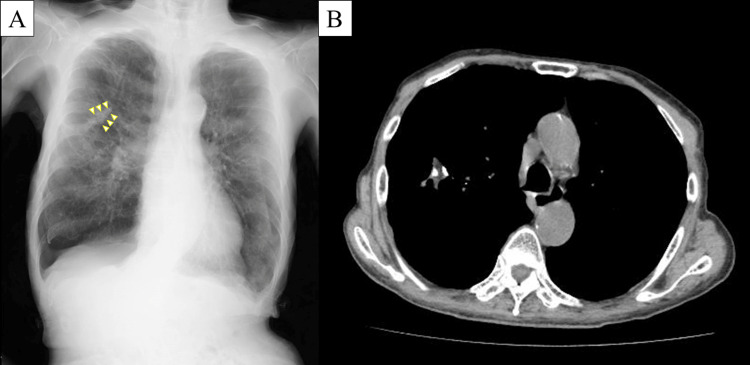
Chest radiograph and computed tomography taken at the time of right pneumothorax recurrence three months after surgery Chest radiograph (A) shows a V-shaped staple line (arrowheads), while computed tomography (B) shows the attached free fat pad had shrunk but remained.
